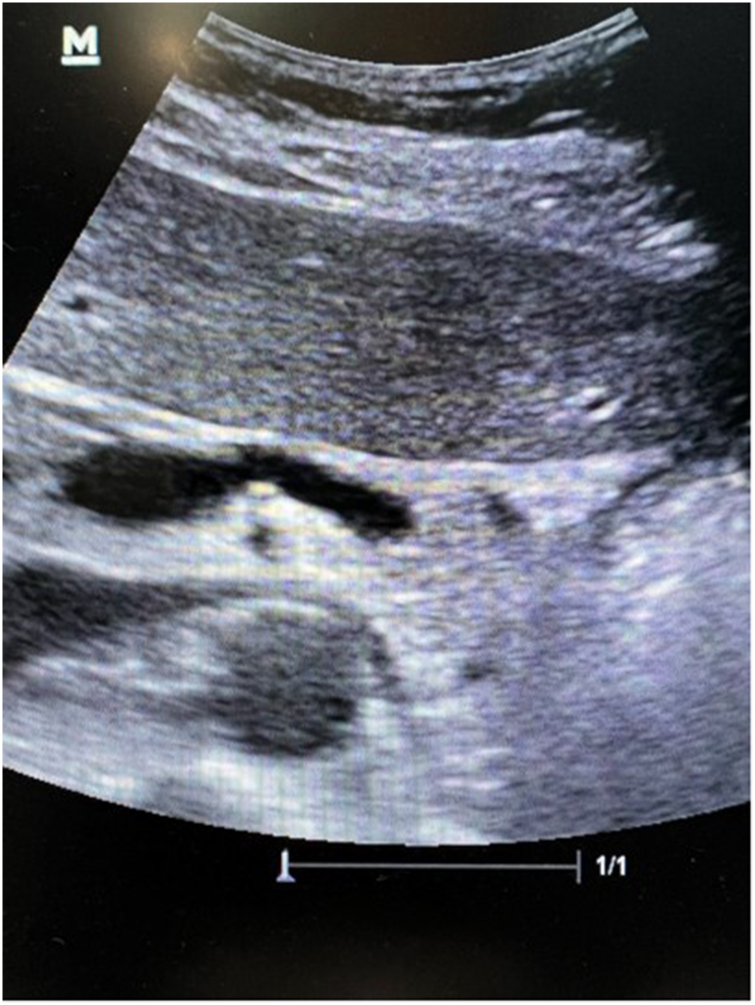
Duplex ultrasonography demonstrating narrowing of the left renal vein between the aorta and superior mesenteric artery
